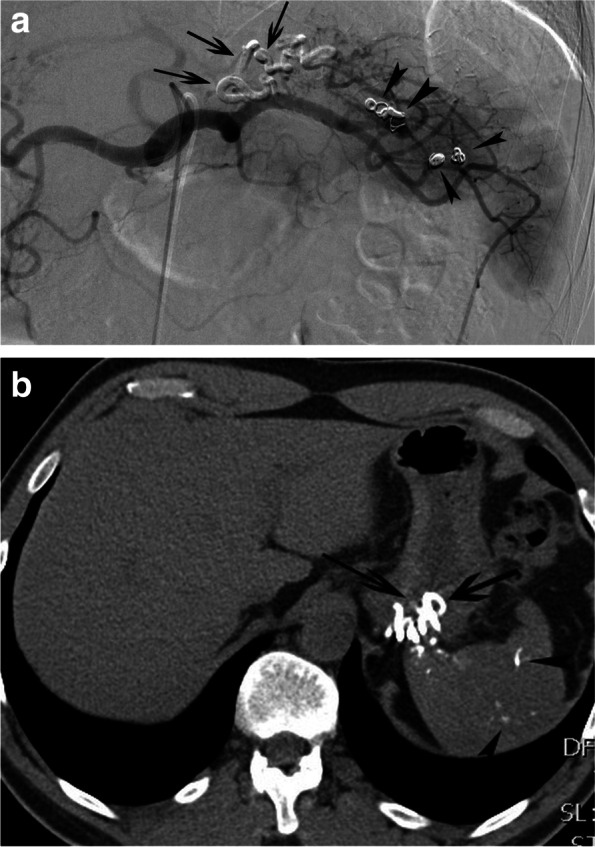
a Selective splenic artery angiography after glue-embolization shows the cast of the glue, completely occluding the cluster of hypertrophied arterial collaterals in the posterior gastric wall. b Follow-up unenhanced CT reveals the dense cast of glue in the cluster of hypertrophied arterial collaterals (black arrows). Note also some droplets of glue (arrowheads), migrated into splenic parenchyma
